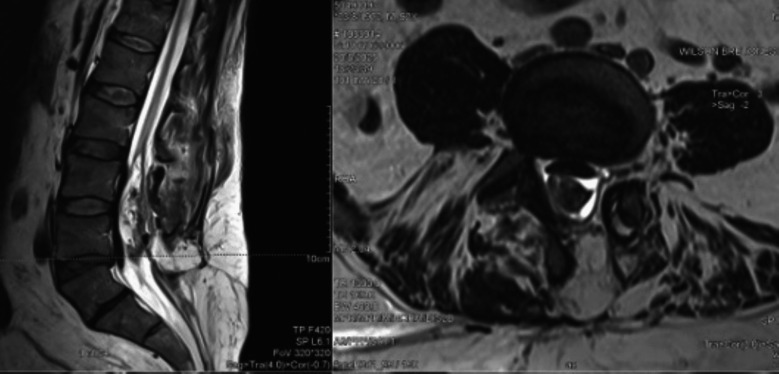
Lumbar spine MRI—postoperative evaluation
